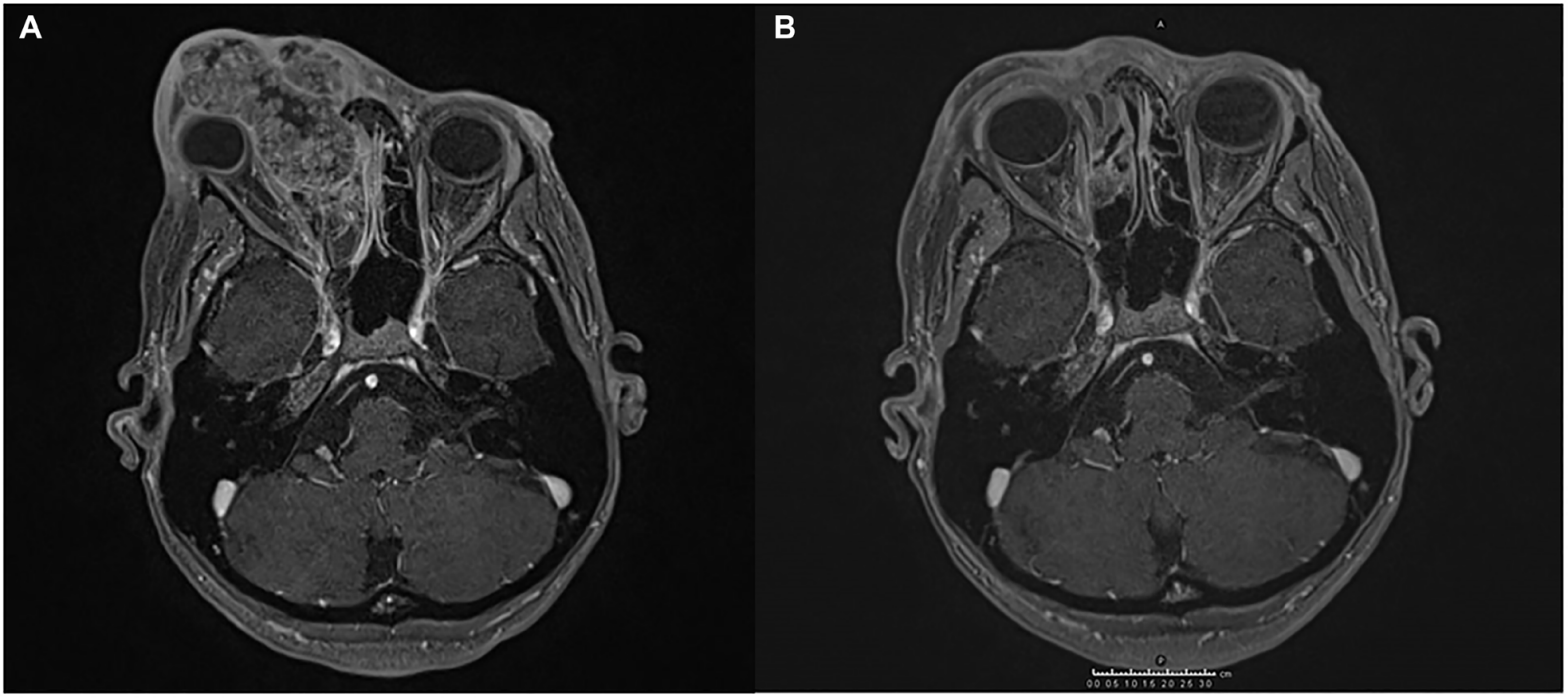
Magnetic resonance imaging (MRI) showing the response of SCC to the treatment administered. (A) An infiltrating lesion engages the medial wall of the right orbital cavity dislocating the eyeball, and invades the right nasal cavity and the nasal septum. (B) The lesion is significantly reduced after hadrontherapy.
